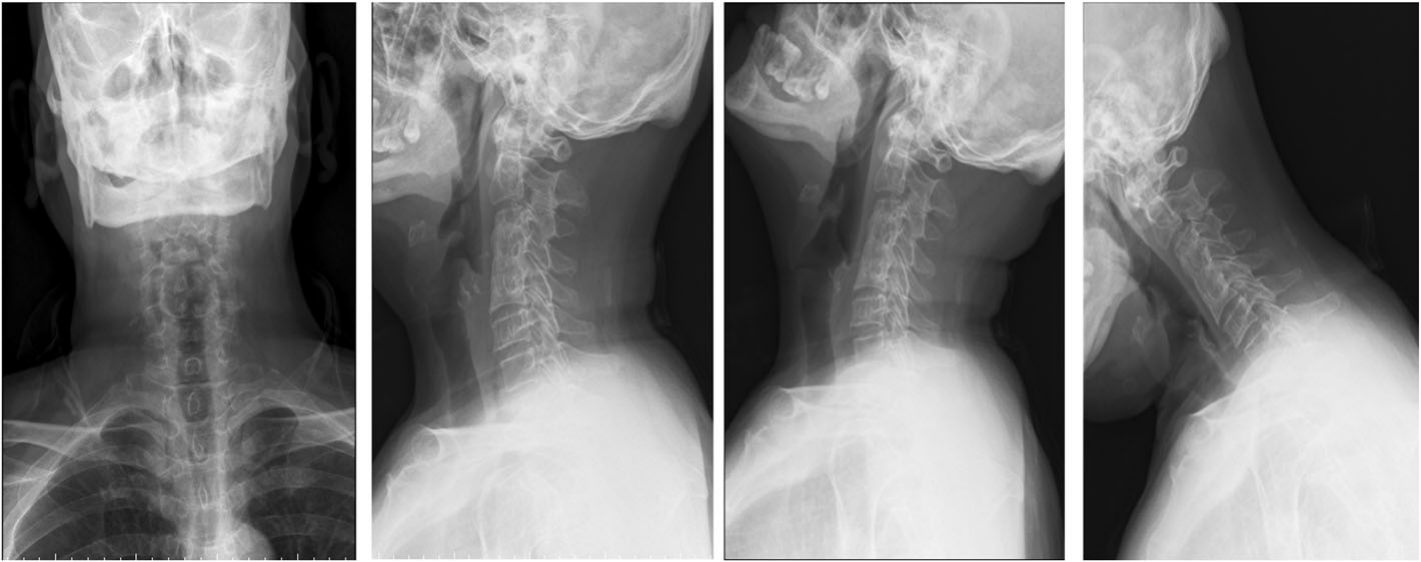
Plain radiograph 11 months after the debridement surgery
